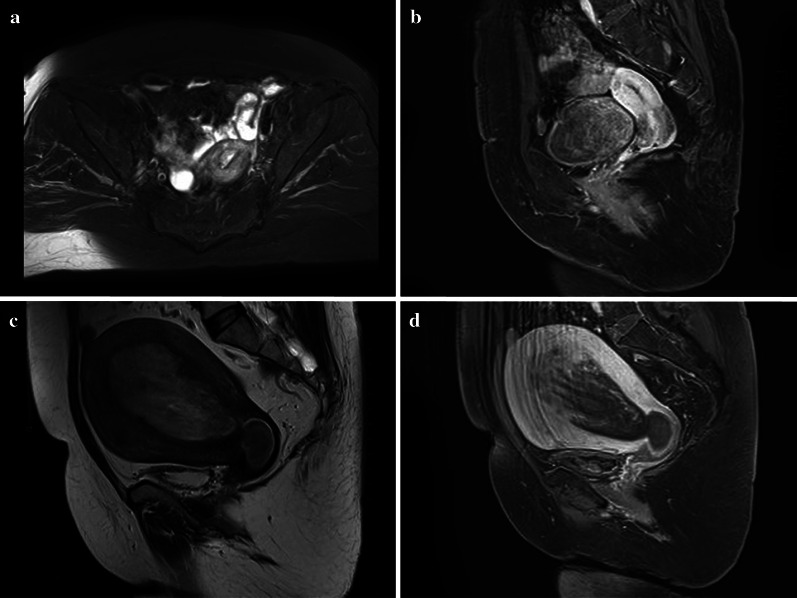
Images of uterine carcinosarcoma (UCS). Axial T2WI magnetic resonance imaging (MRI) (a) of a 63-year-old woman showed uterine fundus endometrial thickening with equal signal. Sagittal delayed enhanced T1WI (b) of the same patient showed a moderate enhancement. Sagittal T2WI MRI (c) of a 55-year-old woman showed a huge mixed signal tumor in the uterine and cervical cavity. Sagittal delayed enhanced T1WI (d) of the same patient showed a heterogeneous enhancement tumor
